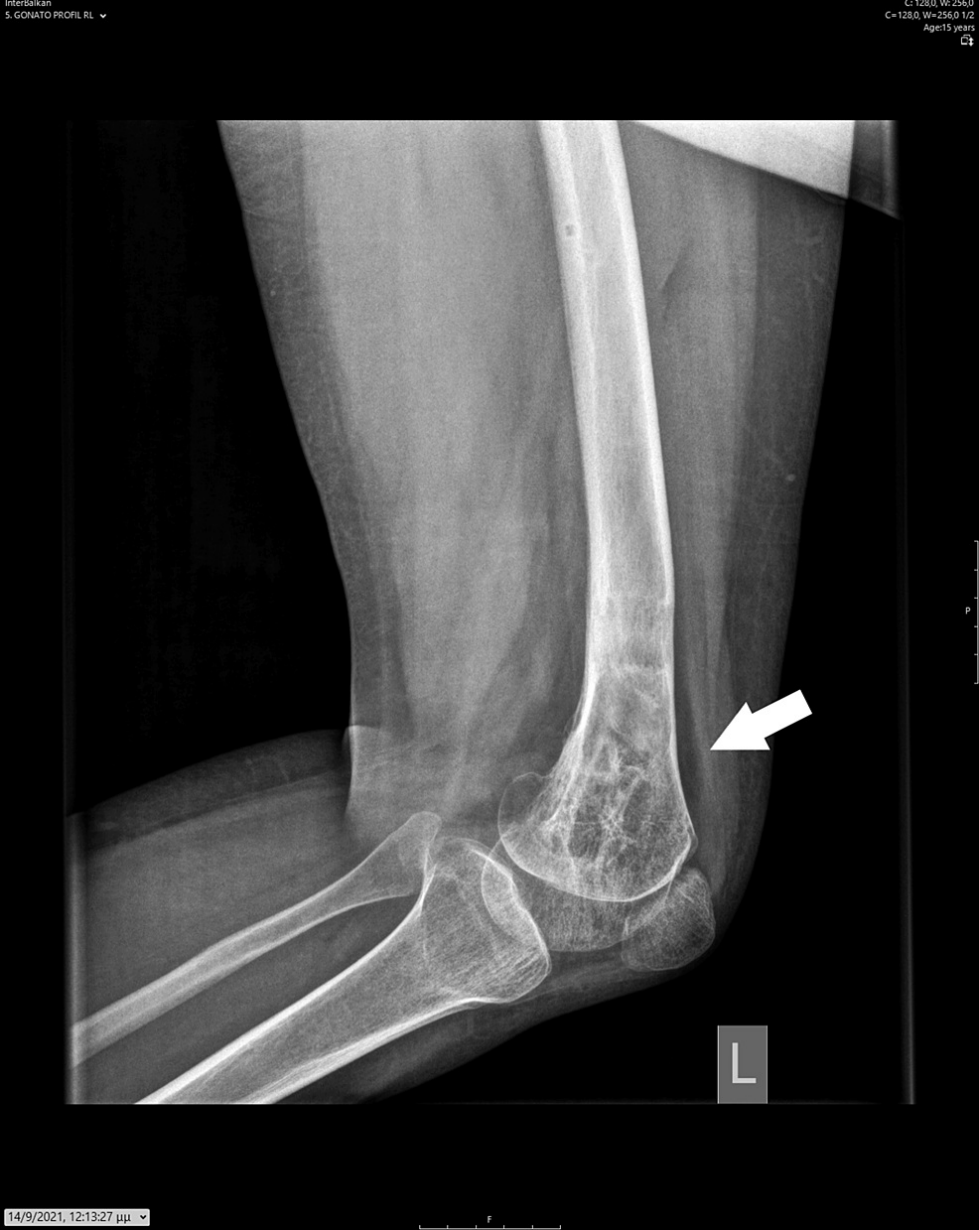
Lateral X-ray of the distal femur, taken one year after the removal of the Ilizarov device.
